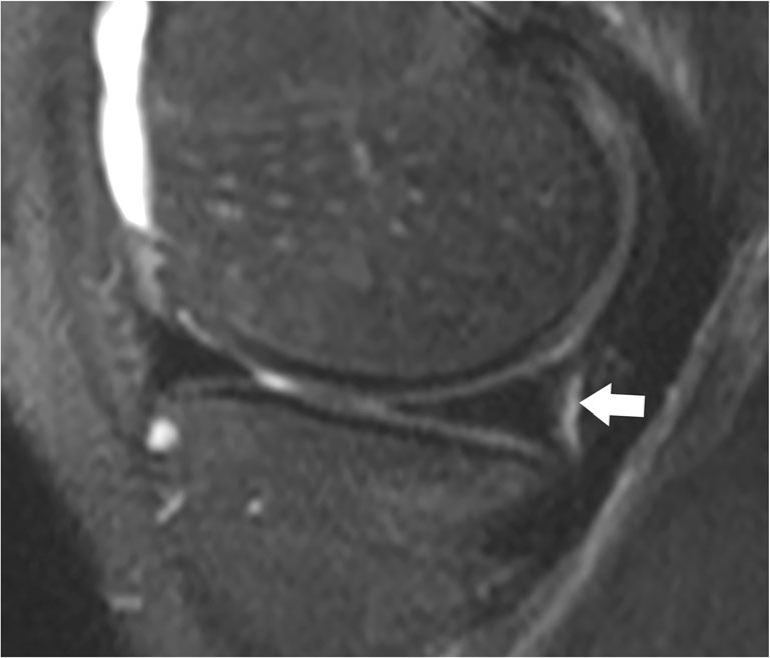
Coronal T2-weighted fat-suppressed fast spin echo image in a 21-year-old male patient shows the normal medial posterior femoral recess or medial gastrocnemius bursa with a small amount of fluid (*arrow*), which should not be diagnosed as a meniscocapsular separation
